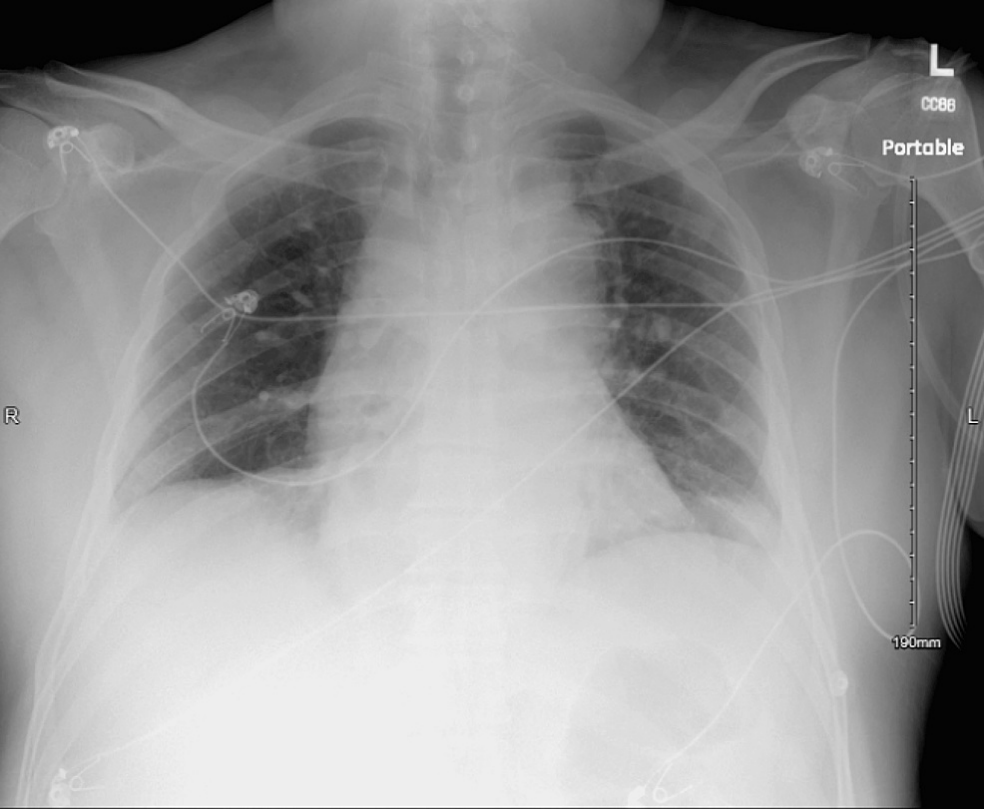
Chest X-ray on postoperative day four showing bilateral pulmonary infiltrates
